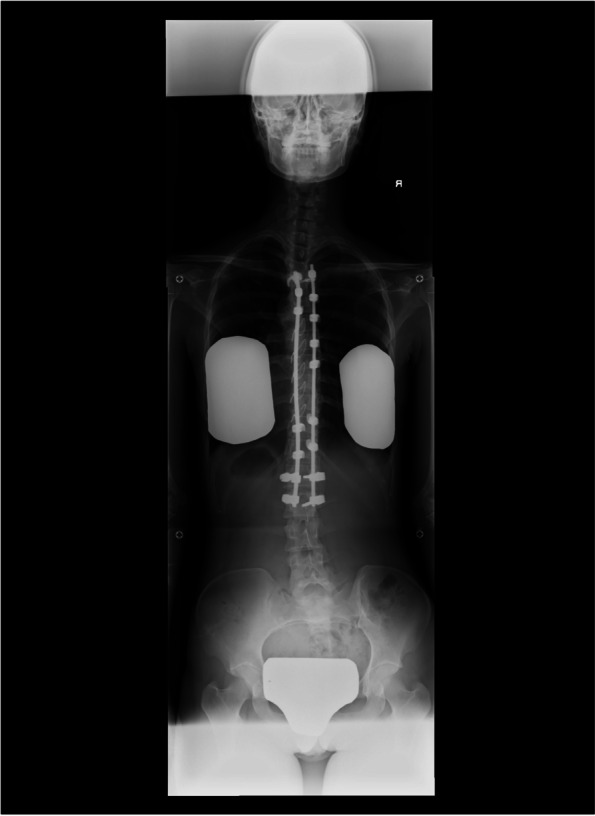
Spinal X-ray for a pregnant woman after fixation surgery. Spinal X-ray of surgical adolescent idiopathic scoliosis demonstrates instrumentation from Th3 to L2
